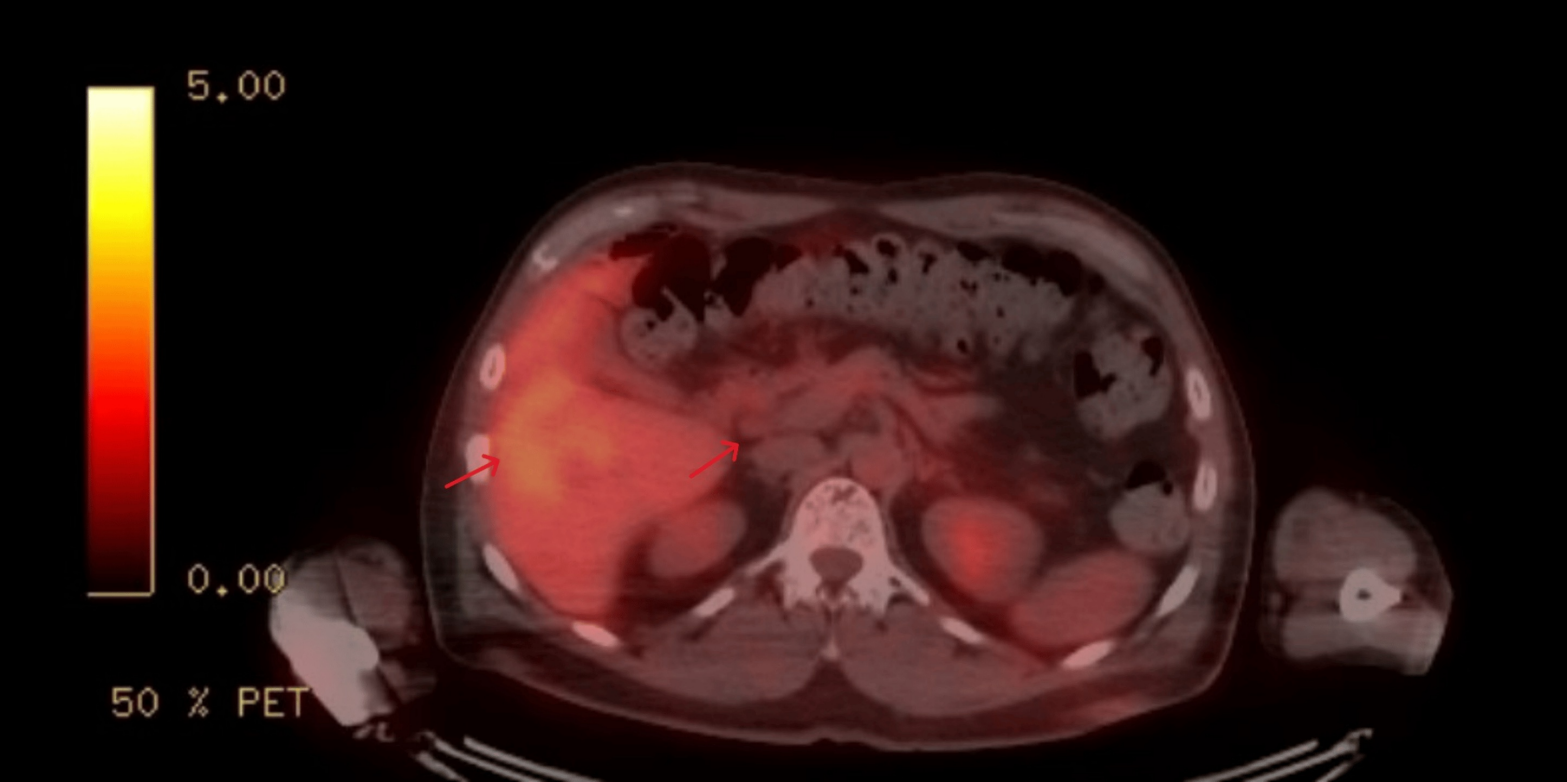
PET-CT after antibiotic therapy showing resolution of liver abnormalities and porta hepatis lymphadenopathy
